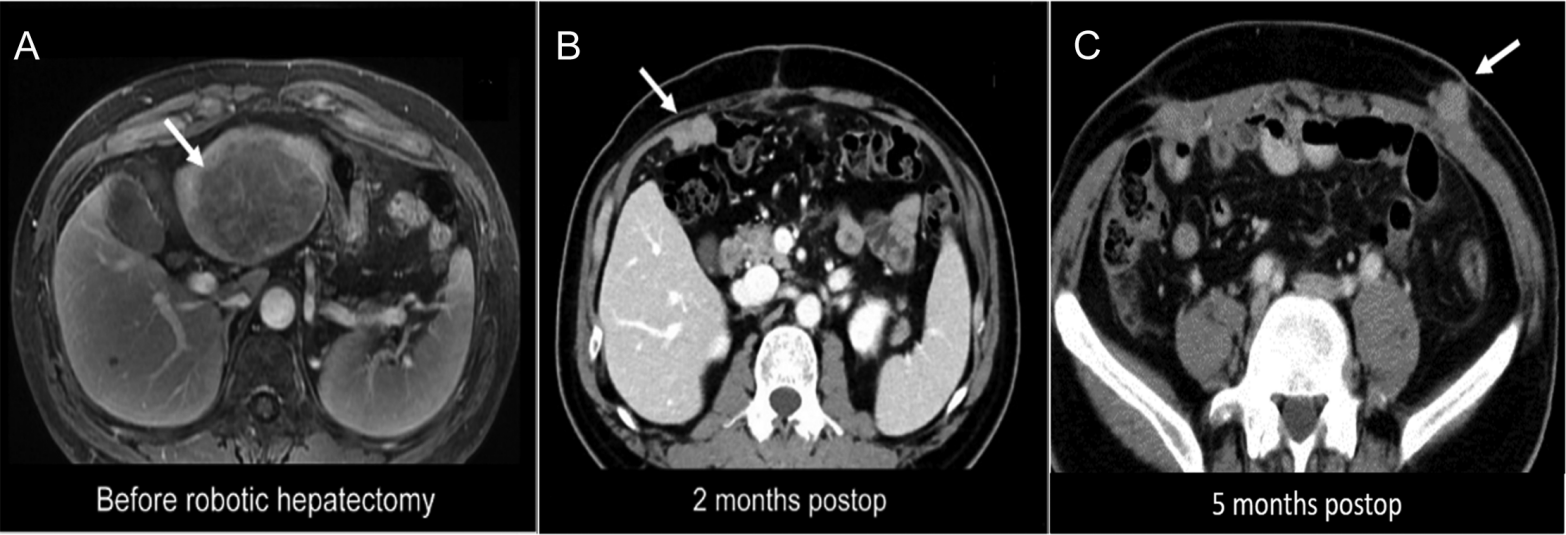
Patient #2 tumor location and late port site metastases. Imaging results for patient #2, who had undergone robotic hepatectomy for a 7.3-cm HCC in S2-3 (A); CT imaging within 2 months postoperatively showed a new 2.8-cm tumor in the right subhepatic region (B); CT imaging within 5 months postoperatively revealed port site metastasis at the left abdominal wall (C).
